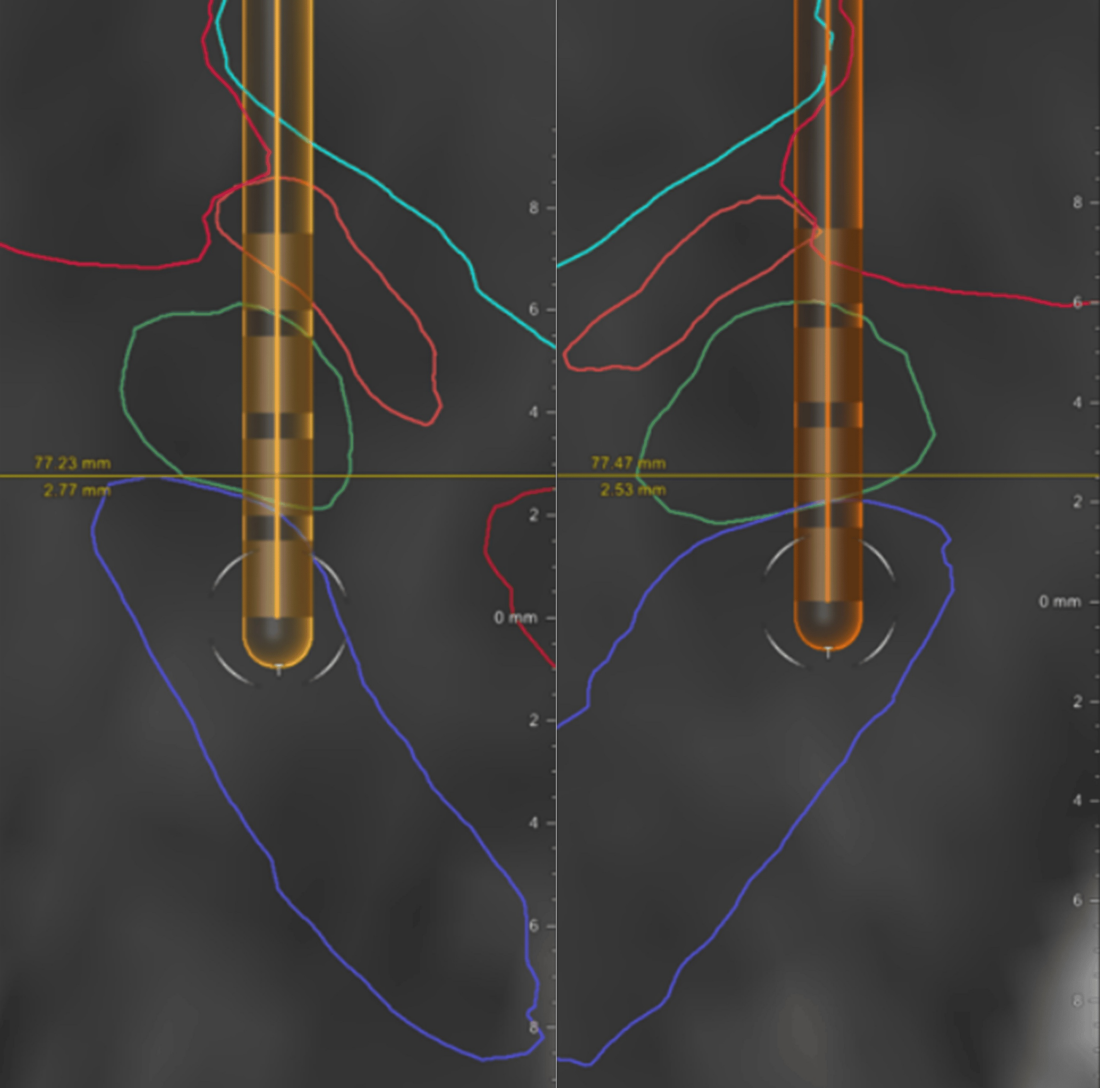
Post-surgical, posterior inline (image plane perpendicular to the electrode trajectory) T2 MRI with spatial reconstruction of left (left) and right (right) DBS leads. The subthalamic nuclei are outlined in green, the substantia nigra in dark blue/purple, the zona incerta in orange, the thalami in light blue, the internal capsules (large structures in top corners) in pink, and the red nucleus in red.
